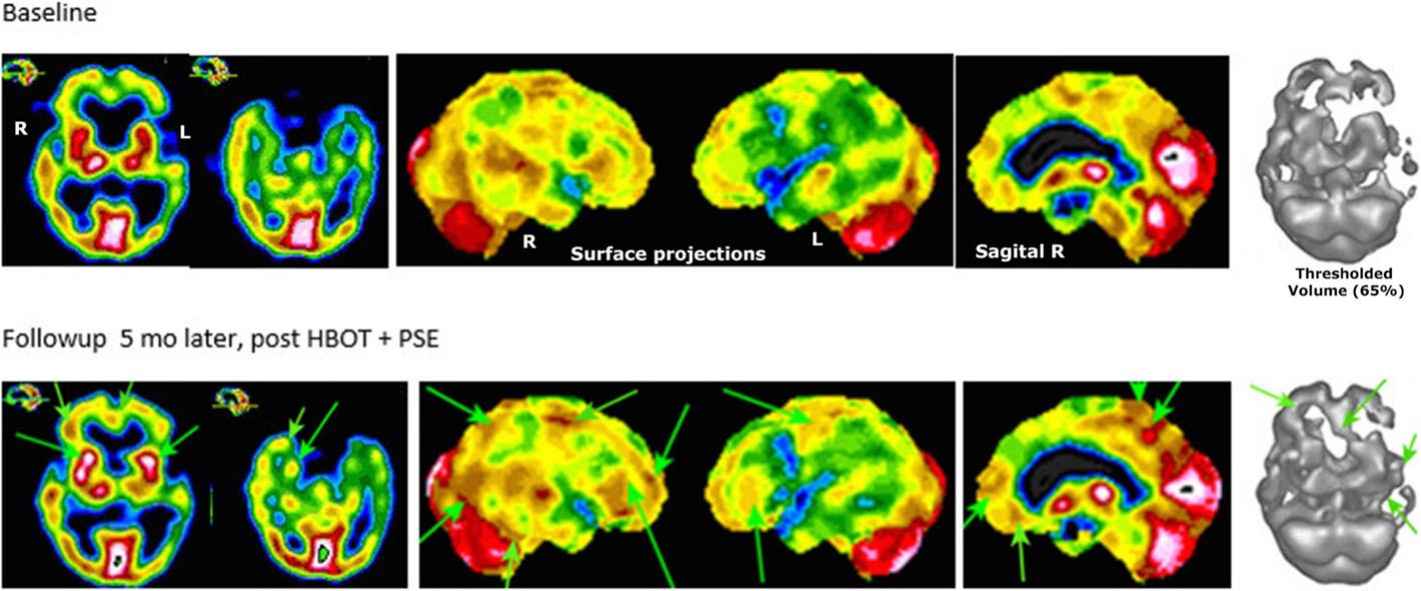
Single-photon emission computed tomography imaging results. The *color code* indicates the level of blood flow, which in turn is proportional to the metabolism level. This enables the detection of hyper or hypo functioning areas: from lowest levels (*blue hues*) to highest levels (*white and black surrounded by white*). The black and white display is a volumetric rendition at a standardized threshold level for both studies. *Upper row*: a baseline single-photon emission computed tomography image revealed extensive left hemispheric underperfusion, and multiple localized underperfusions in the right hemisphere. Bilateral involvement of parts of the dorsolateral prefrontal cortex, and extensive underperfusion in the temporal lobes (more on the left) and to a lesser extent in the orbitofrontal areas. *Lower row*: a follow-up single-photon emission computed tomography image after 5 months showed similar overall appearance but with increased perfusion in multiple small localized areas namely in parts of the anterior aspect of the prefrontal cortex (including in the ventromesial aspect), right superior parietal, right lateral occipital, superior aspect of the left frontoparietal area, posterior cingulate/precuneus and apico-mesial right temporal. In addition, significant increase was seen in the striatum bilaterally. *HBOT* hyperbaric oxygen therapy, *PSE* perispinal etanercept
